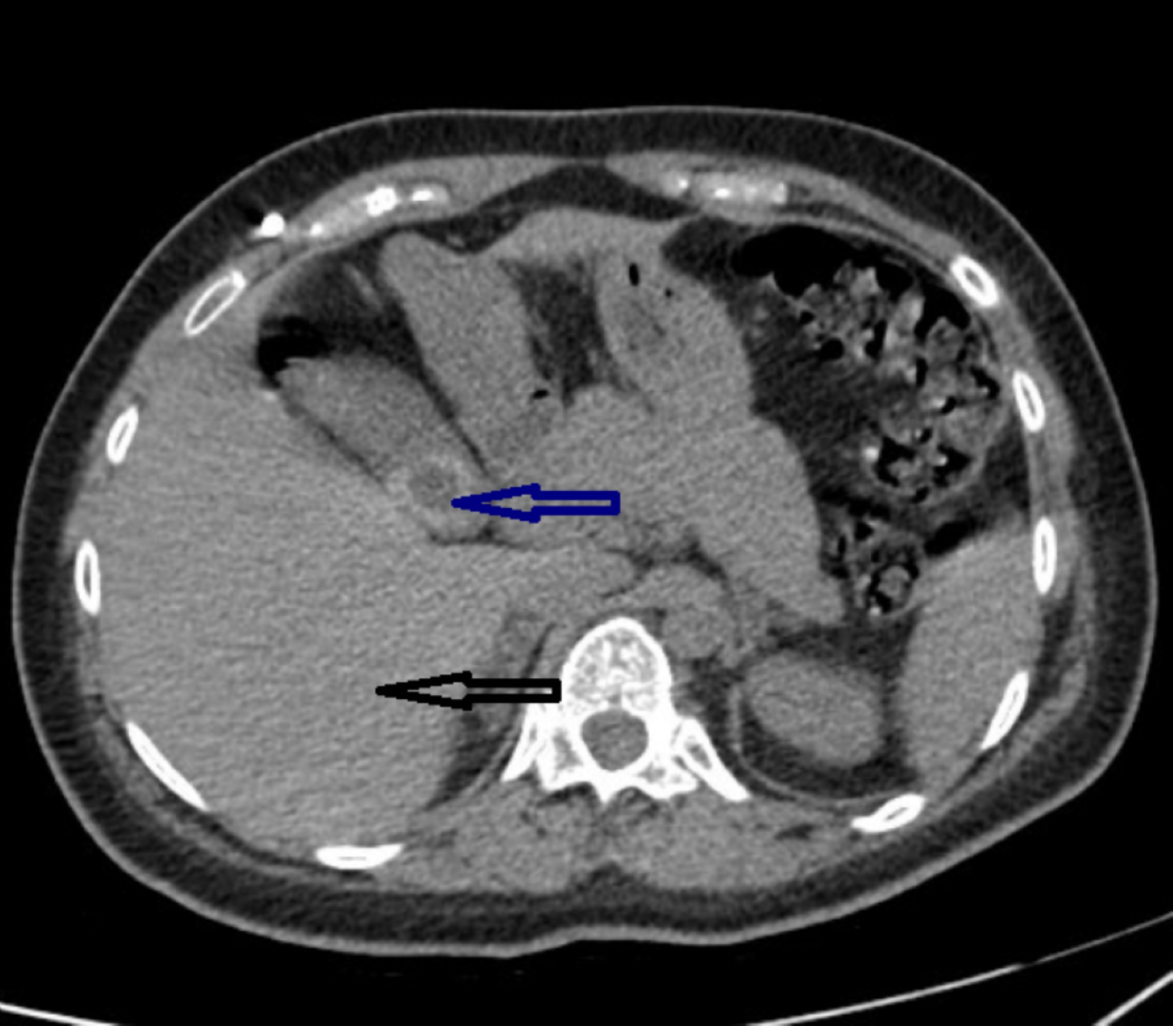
CT of abdomen Black arrow demonstrates normal, non-cirrhotic liver; Blue arrow demonstrates gallstones CT: computed tomography
